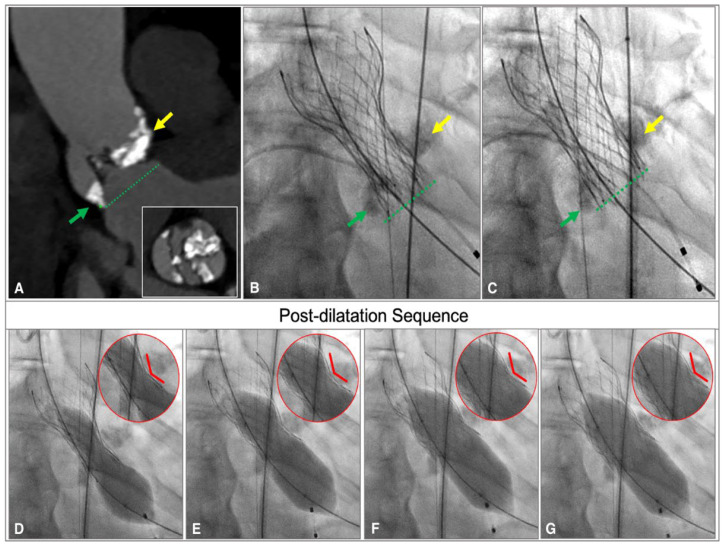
CT and Angiographic view of a Corevalve Evolut FX with a “K” shape at deployment and its post-dilatation sequence in BAV anatomy. Long-axis and short-axis CT scans show a raphe-type BAV where green arrow indicates VBR plane and yellow arrow indicates the LIRA plane (A); angiographic view of a Corevalve Evolut FX with two prevalent points of constraints corresponding to VBR plane (green arrow) and LIRA plane (yellow arrow) before pre-dilatation (B) and after post-dilatation (C); effective post-dilatation sequence (D–G) of a highly constrained prosthesis with persistence of the waist and the K shape (red circle).
